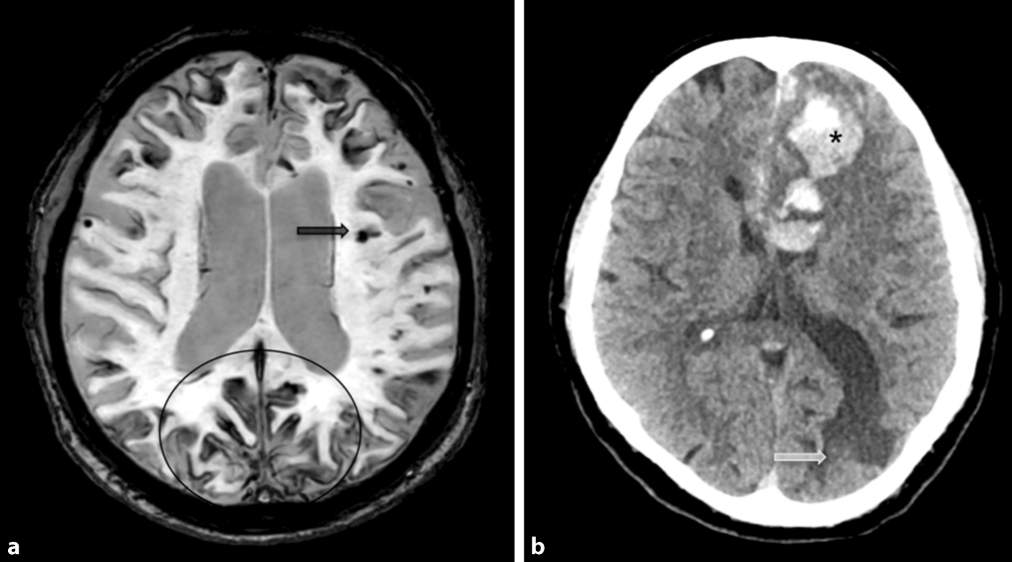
Cerebral amyloid angiopathy. A 60-year-old female patient with cerebral amyloid angiopathy who presented with superficial hemosiderosis, particularly frontal and occipital (the latter marked with a circle), as well as multiple microbleeds (*black arrow*) representing hemosiderin deposits on axial susceptibility-weighted images (a). There was also an acute intracranial bleeding in the left frontal lobe (*Asterisk*), as well as a post-hemorrhagic parenchymal defect in the left occipital lobe (*white arrow*) on axial computed tomography images (b)
